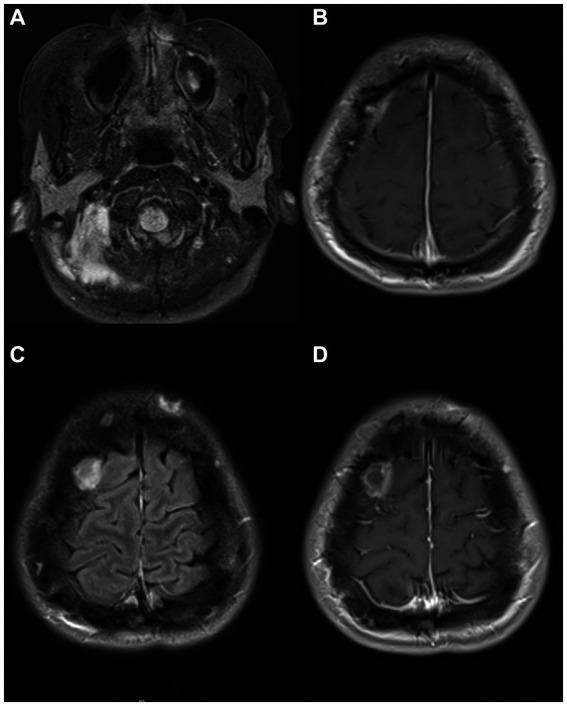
MRI images from a 31-year-old woman with anti-IFN-γ autoantibodies and *Mycobacterium avium* infection. Brain MRI images showing a left post-auricular lesion with erosion through the bone [(A) – postcontrast FLAIR] and a right frontal epidural collection [(B) – postcontrast T1], later also extending into right frontal parenchyma [(C) – postcontrast FLAIR, (D)– postcontrast T1]. FLAIR, fluid-attenuated inversion recovery; IFN, interferon.
